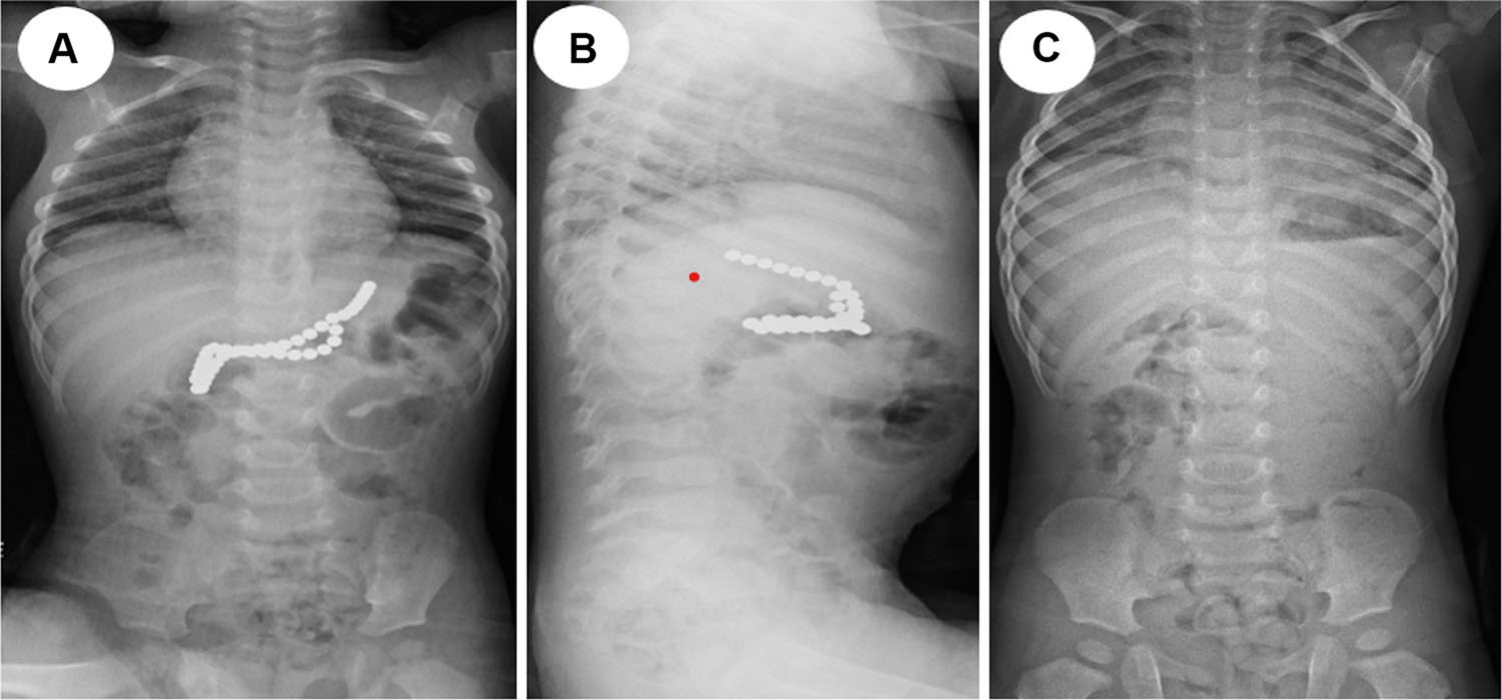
Radiograph showing 40 magnets removed endoscopically from stomach and duodenum. (A) AP view; (B) Lateral view; (C) after removal of magnets.
